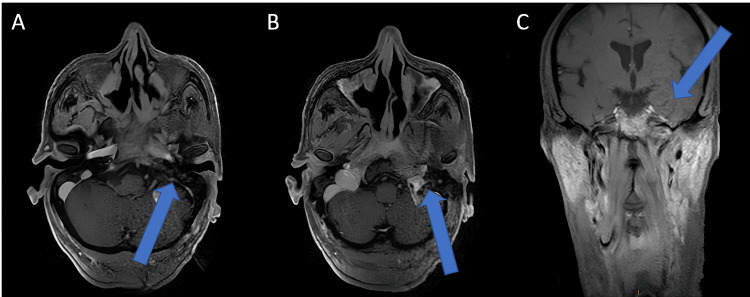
Post-treatment contrast-enhanced T1 sequence angio-MRI of the nasopharynx A, B: axial views showing a reduction in tumor size (arrow) to approximately 30 × 26 mm, with disappearance of invasion of the left infratemporal fossa; C: coronal view showing resolution of the intracranial extension (arrow).
